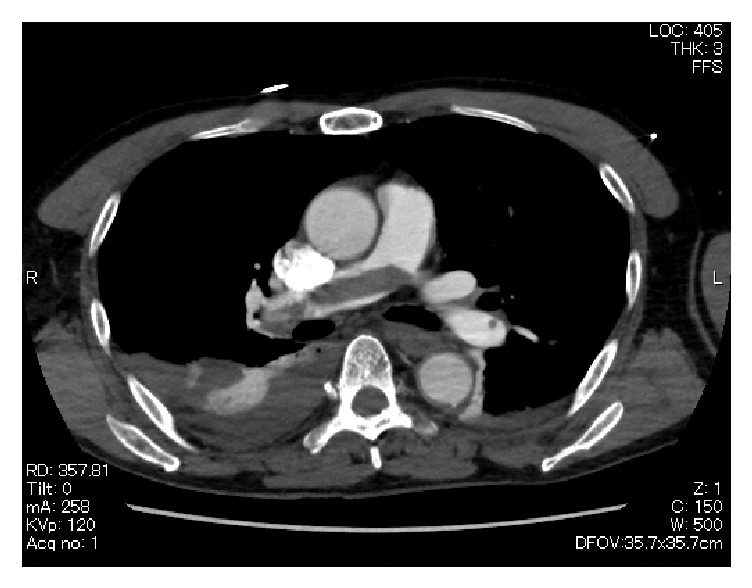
Enhanced computed tomography revealing a pulmonary embolism (white arrow).
